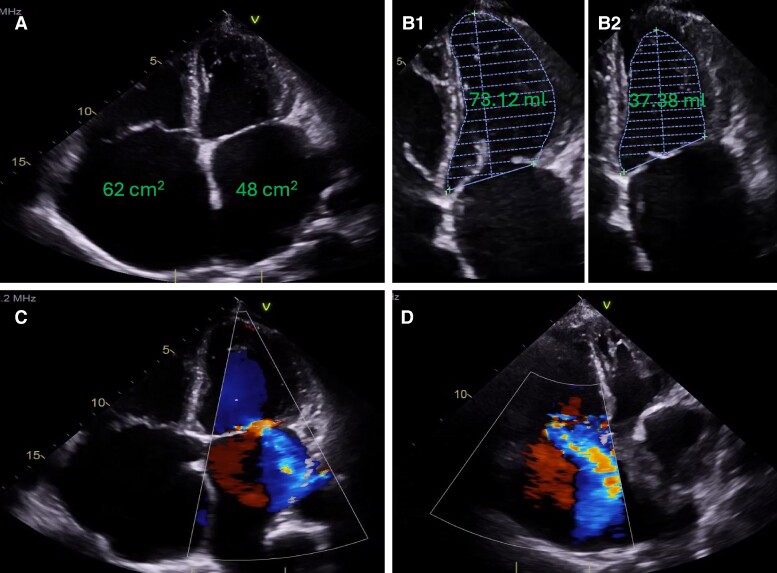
Transoesophageal echocardiogram at 20-year follow-up. Bi-atrial dilatation with atrial volumes (*A*), left ventricular function [end-diastolic volume (*B.1*), end-systolic volume (*B.2*)], mitral regurgitation (*C*), and tricuspid regurgitation (*D*).
